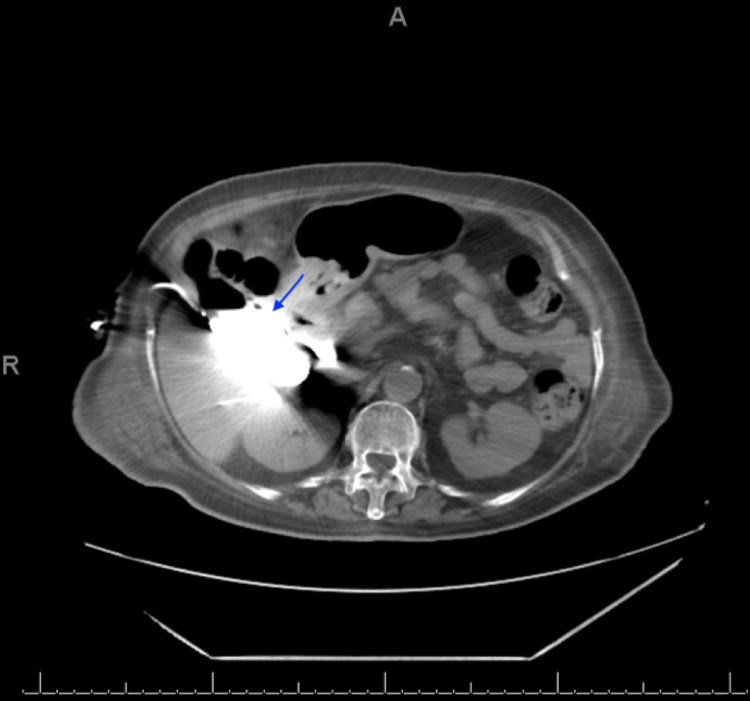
CT scan of the abdomen without intravenous contrast. The contrast was injected through the cholecystostomy tube. CT: computed tomography
